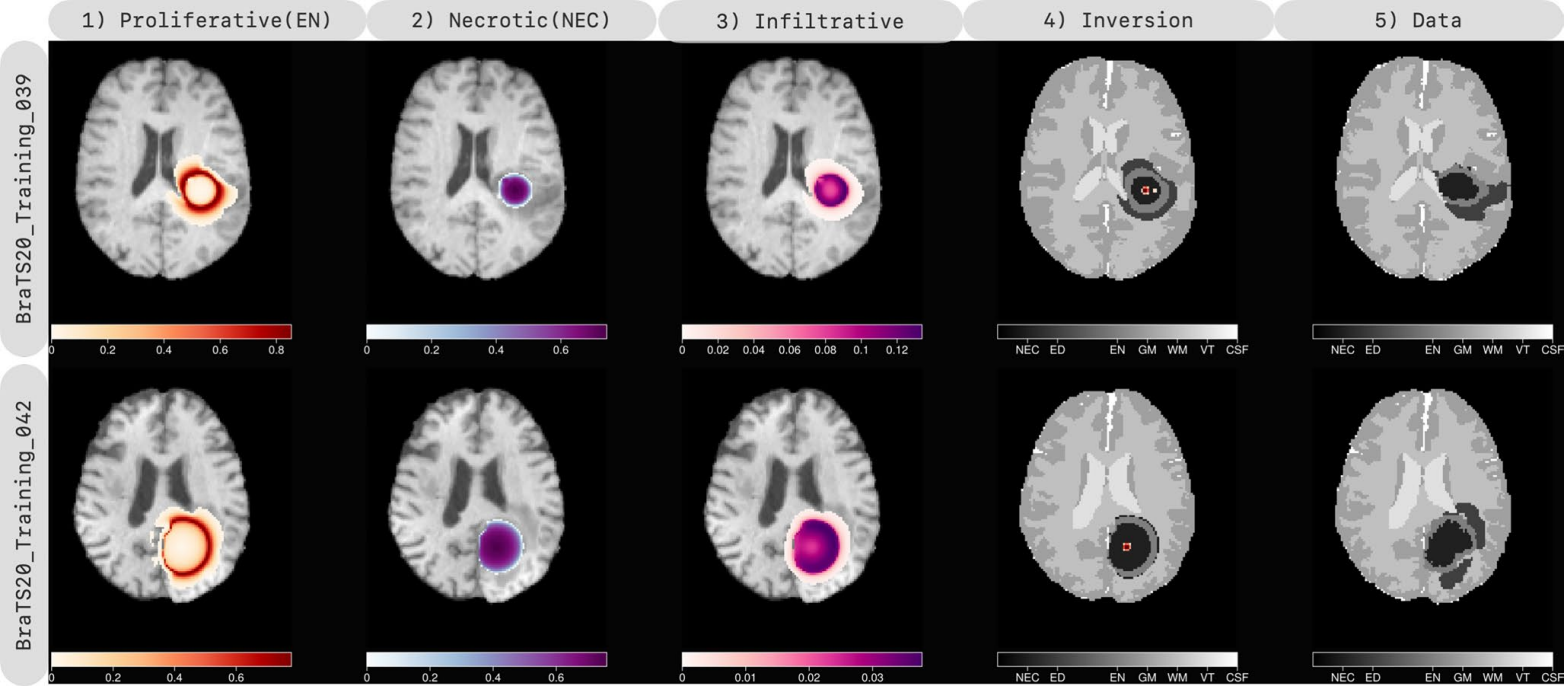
We conduct a multi‐species inversion of the BraTS20_Training clinical dataset, selecting two patients, using the MRI scan segmentation as input. Our results are presented in a tabular format, with each row showing the inversion and data for each patient. The first three columns depict the inverted species (proliferative or enhancing (EN), necrotic (NEC), and infiltrative cells), the fourth column shows the observed species from the inversion, and the last column displays the data. Our results demonstrate that, despite only having a single snapshot from each patient, we can qualitatively reconstruct the species distribution with reasonable accuracy.
